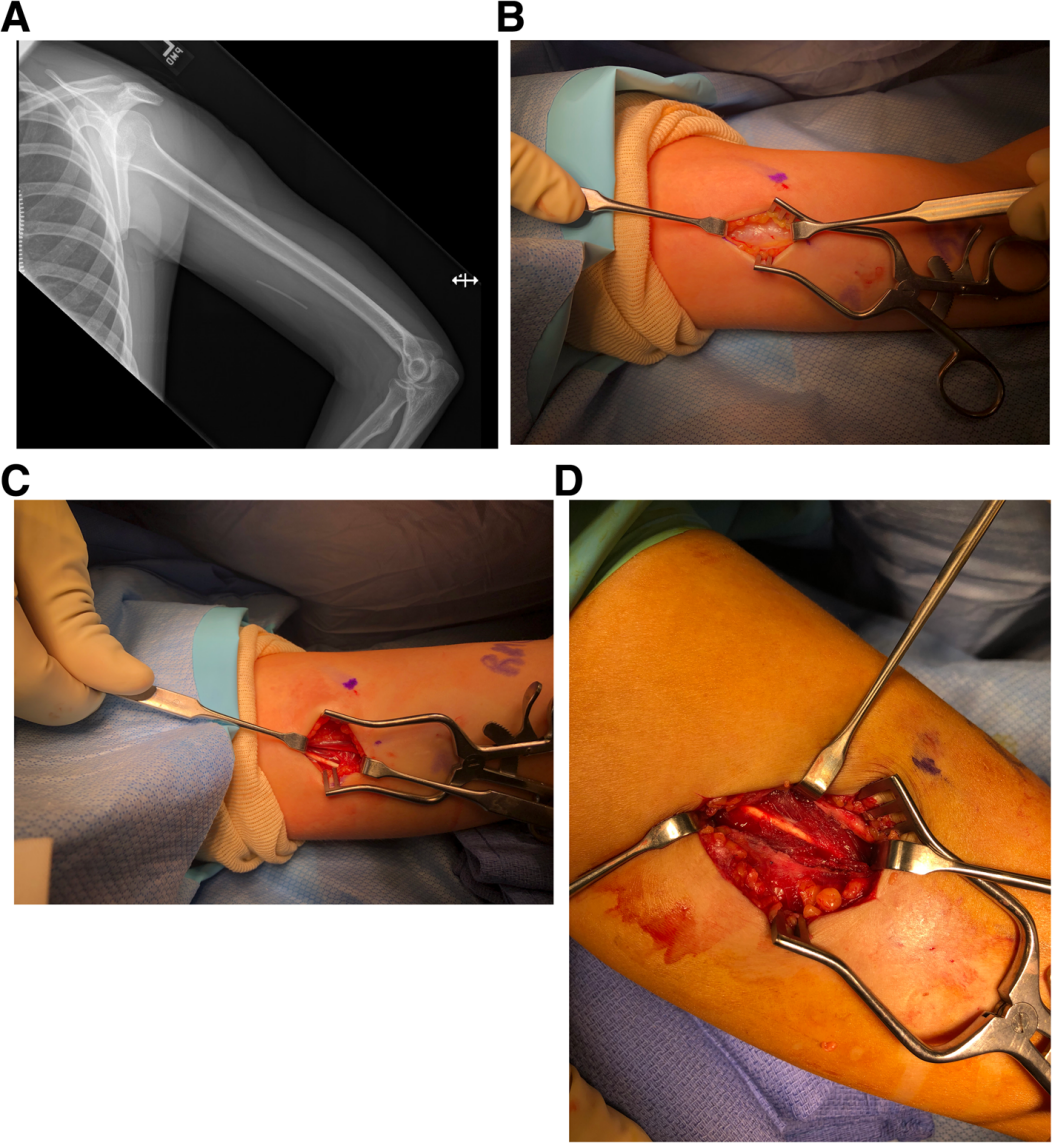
Radiographic identification of implanted contraceptive implant prior to incision in operating room (a). Incision and blunt dissection carried down to the fascial layer of the biceps brachii, which must be incised to retrieve the implant (b). Etonogestrel implant located deep to the biceps brachii fascia (c). Etonogestrel implant located within the muscle belly of the biceps brachii, surrounded by muscle fibers (d)
